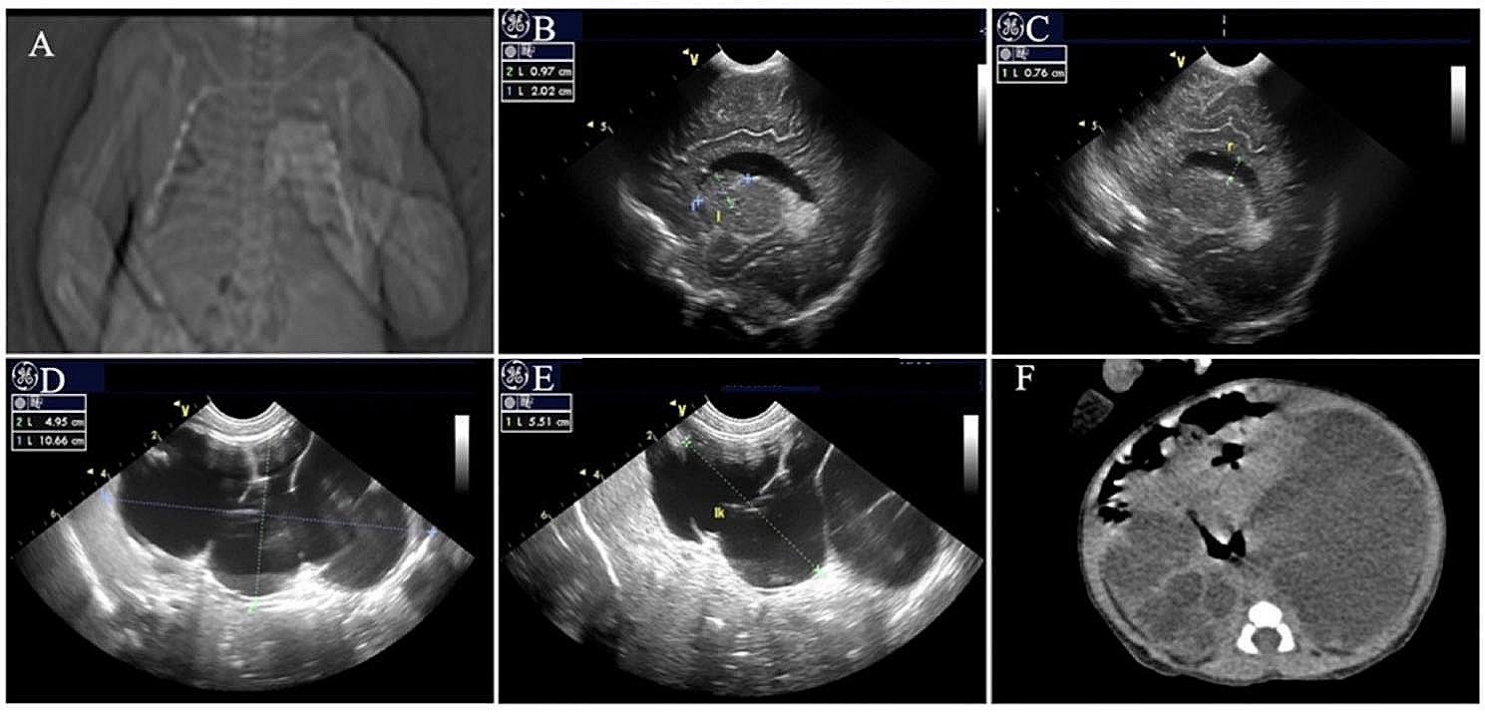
Photographs show the clinical features of the patient in this study. (A) Chest radiographs at 1 day after birth show wide ribsn (B, C) Color doppler ultrasonography at 10 days after birth shows widened bodies and anterior horns of lateral ventricles; (D, E) Color doppler ultrasonography at 10 days after birth shows severe hydronephrosis was found in both kidneys, bilateral pelvis and calyces were severely dilated with a “palette” appearancete (F) Abdominal CT at 1 day after birth shows Significant increase in the size of both kidneys, signs of severe hydronephrosis in both kidneys
